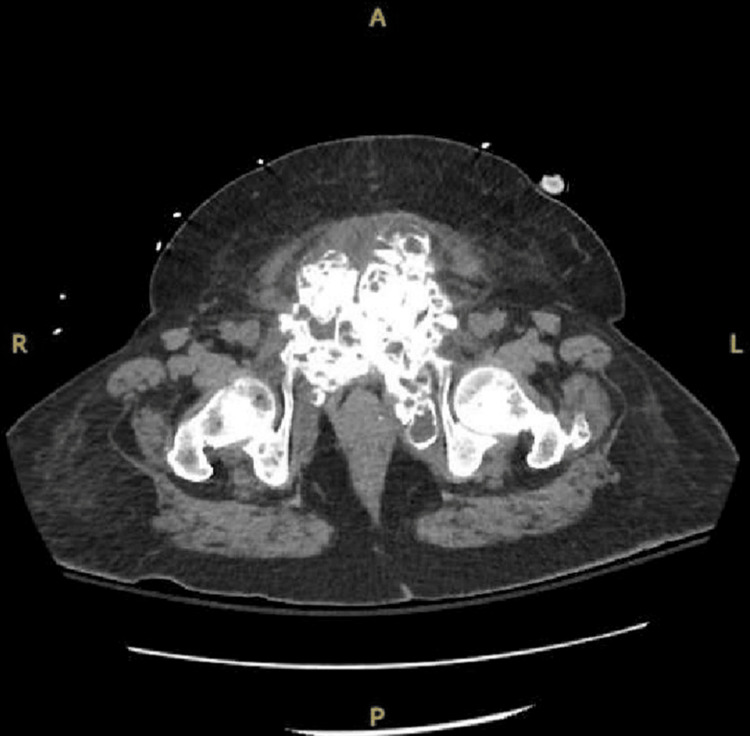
CT axial view of the abdomen and pelvis redemonstrates uremic tumoral calcinosis of the pubic symphysis, with the left side worse than the right in posterior extension.
